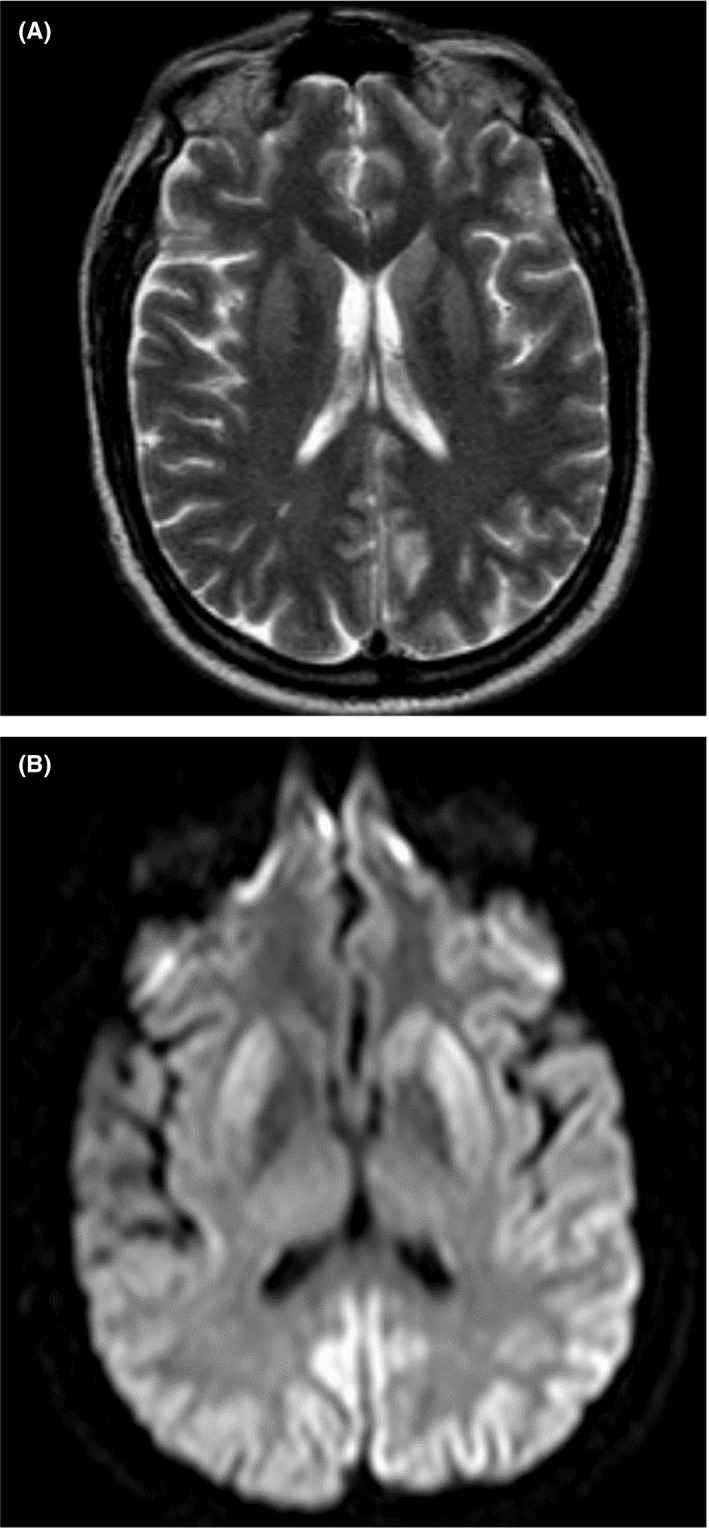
A, MRI abnormal signal density on bilateral caudate and basal ganglia on DWI. B, MRI abnormal signal density on bilateral caudate and basal ganglia on T2
